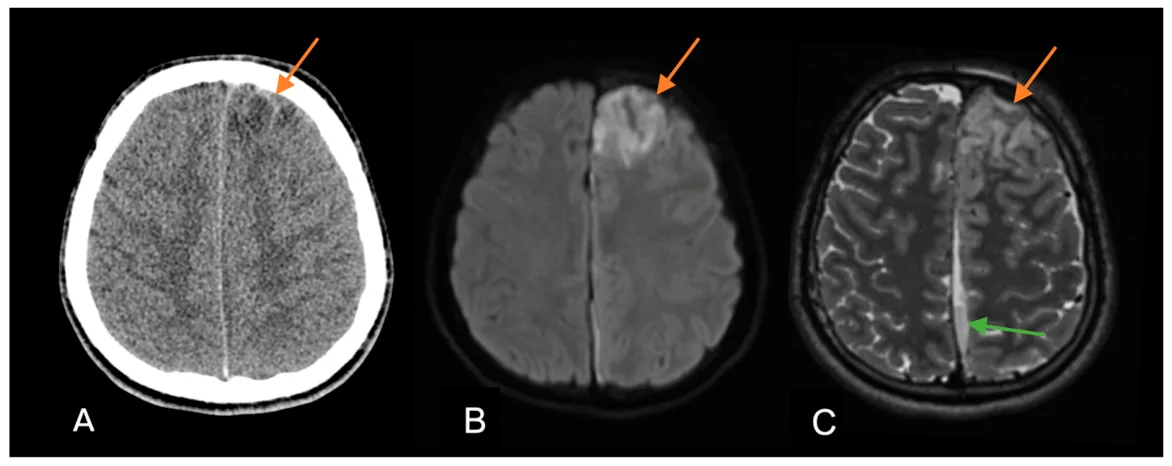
Initial cranial imaging. (A) Native cranial CT—the arrow shows a left frontal hypodense lesion with hemorrhagic components. (B) Axial diffusion-weighted MRI—the arrow shows left frontopolar cerebritis and restricted diffusion compatible with purulent intracranial infection. (C) Axial T2-weighted MRI—the orange arrow shows left frontal parenchymal hyperintensity and the green arrow shows an adjacent parafalcine/subdural empyema.
